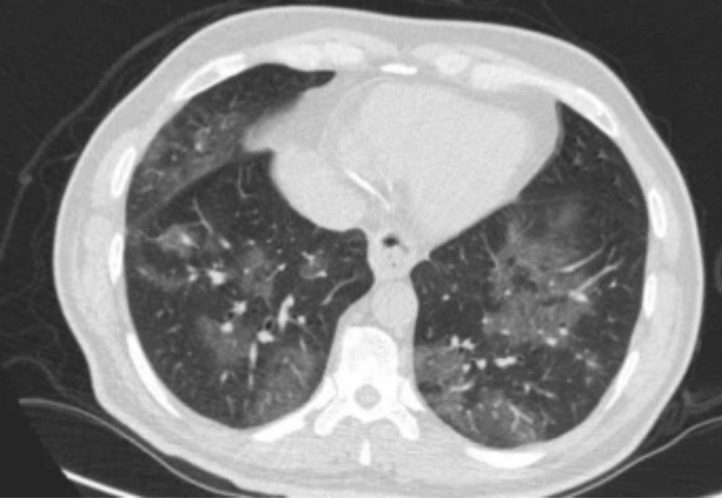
COVID-19 pneumonia: section CT shows bilateral multifocal subpleural and GGO
